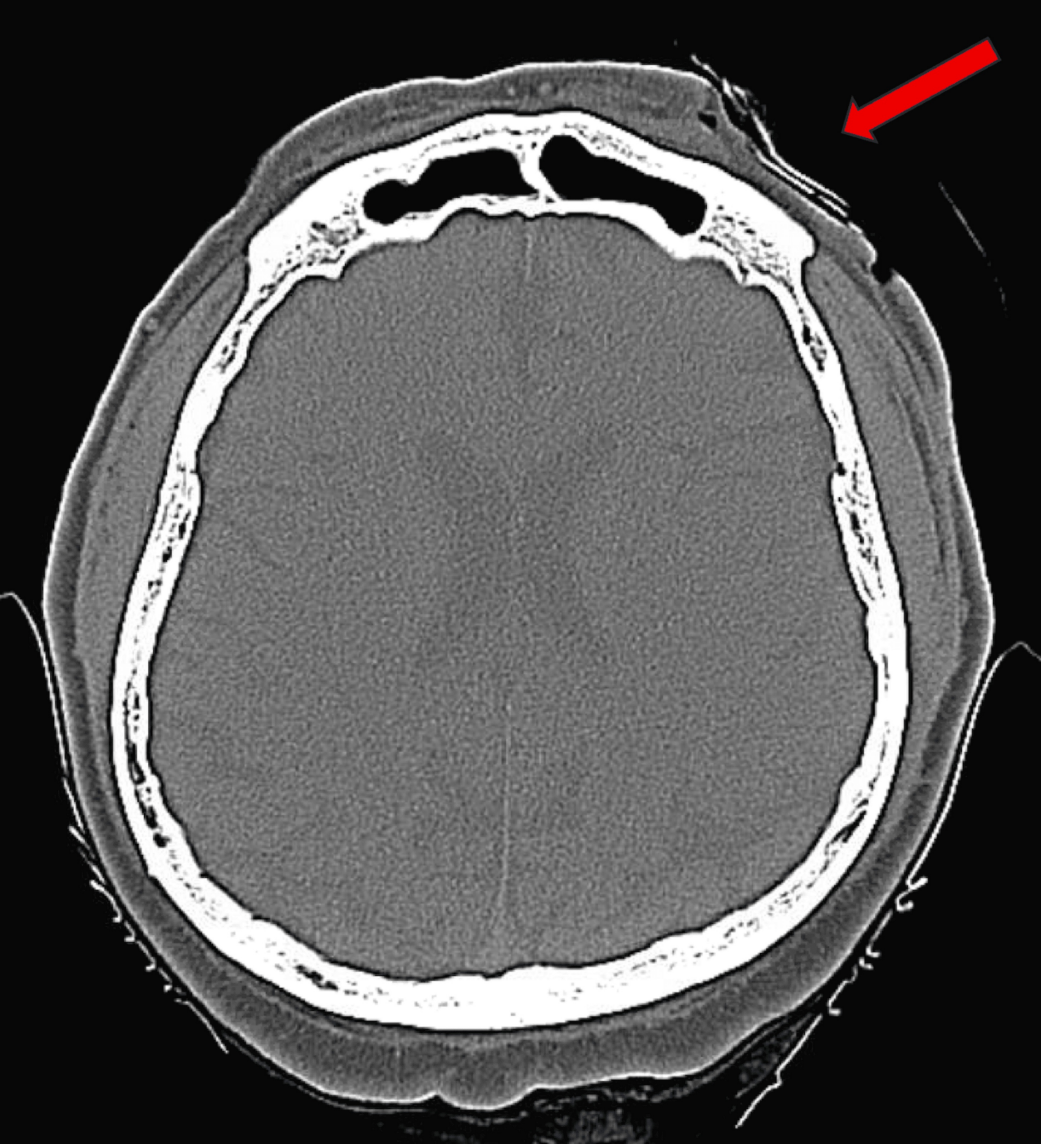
Axial computed tomography in the bone window at the level of the left eyebrow. The region of interest (red arrow) shows the intact bone and normal soft tissue without evidence of a residual tumor.
